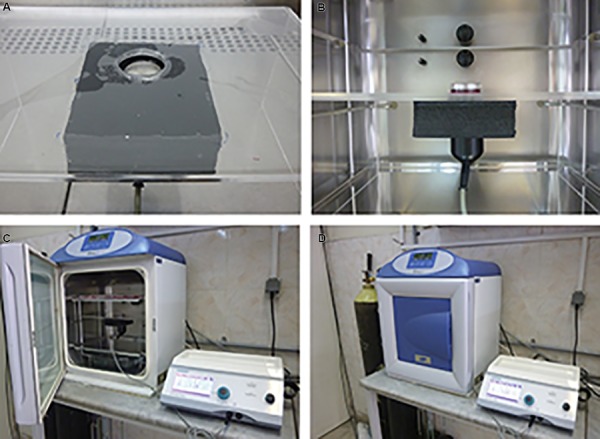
Experimental set-up of the ultrasound-mediated spermatogonial stem cells (SSCs) stimulation. A, B. Position of ultrasound transducer and cell
